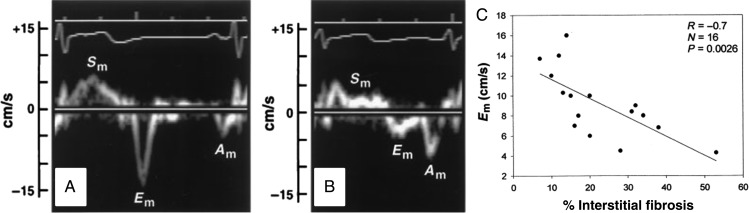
Tissue Doppler in normal *(A)* and chronically ischaemic dysfunctional myocardium *(B)*: E’ is related to the degree of interstitial fibrosis adapted from Shan *et al.*^144^
